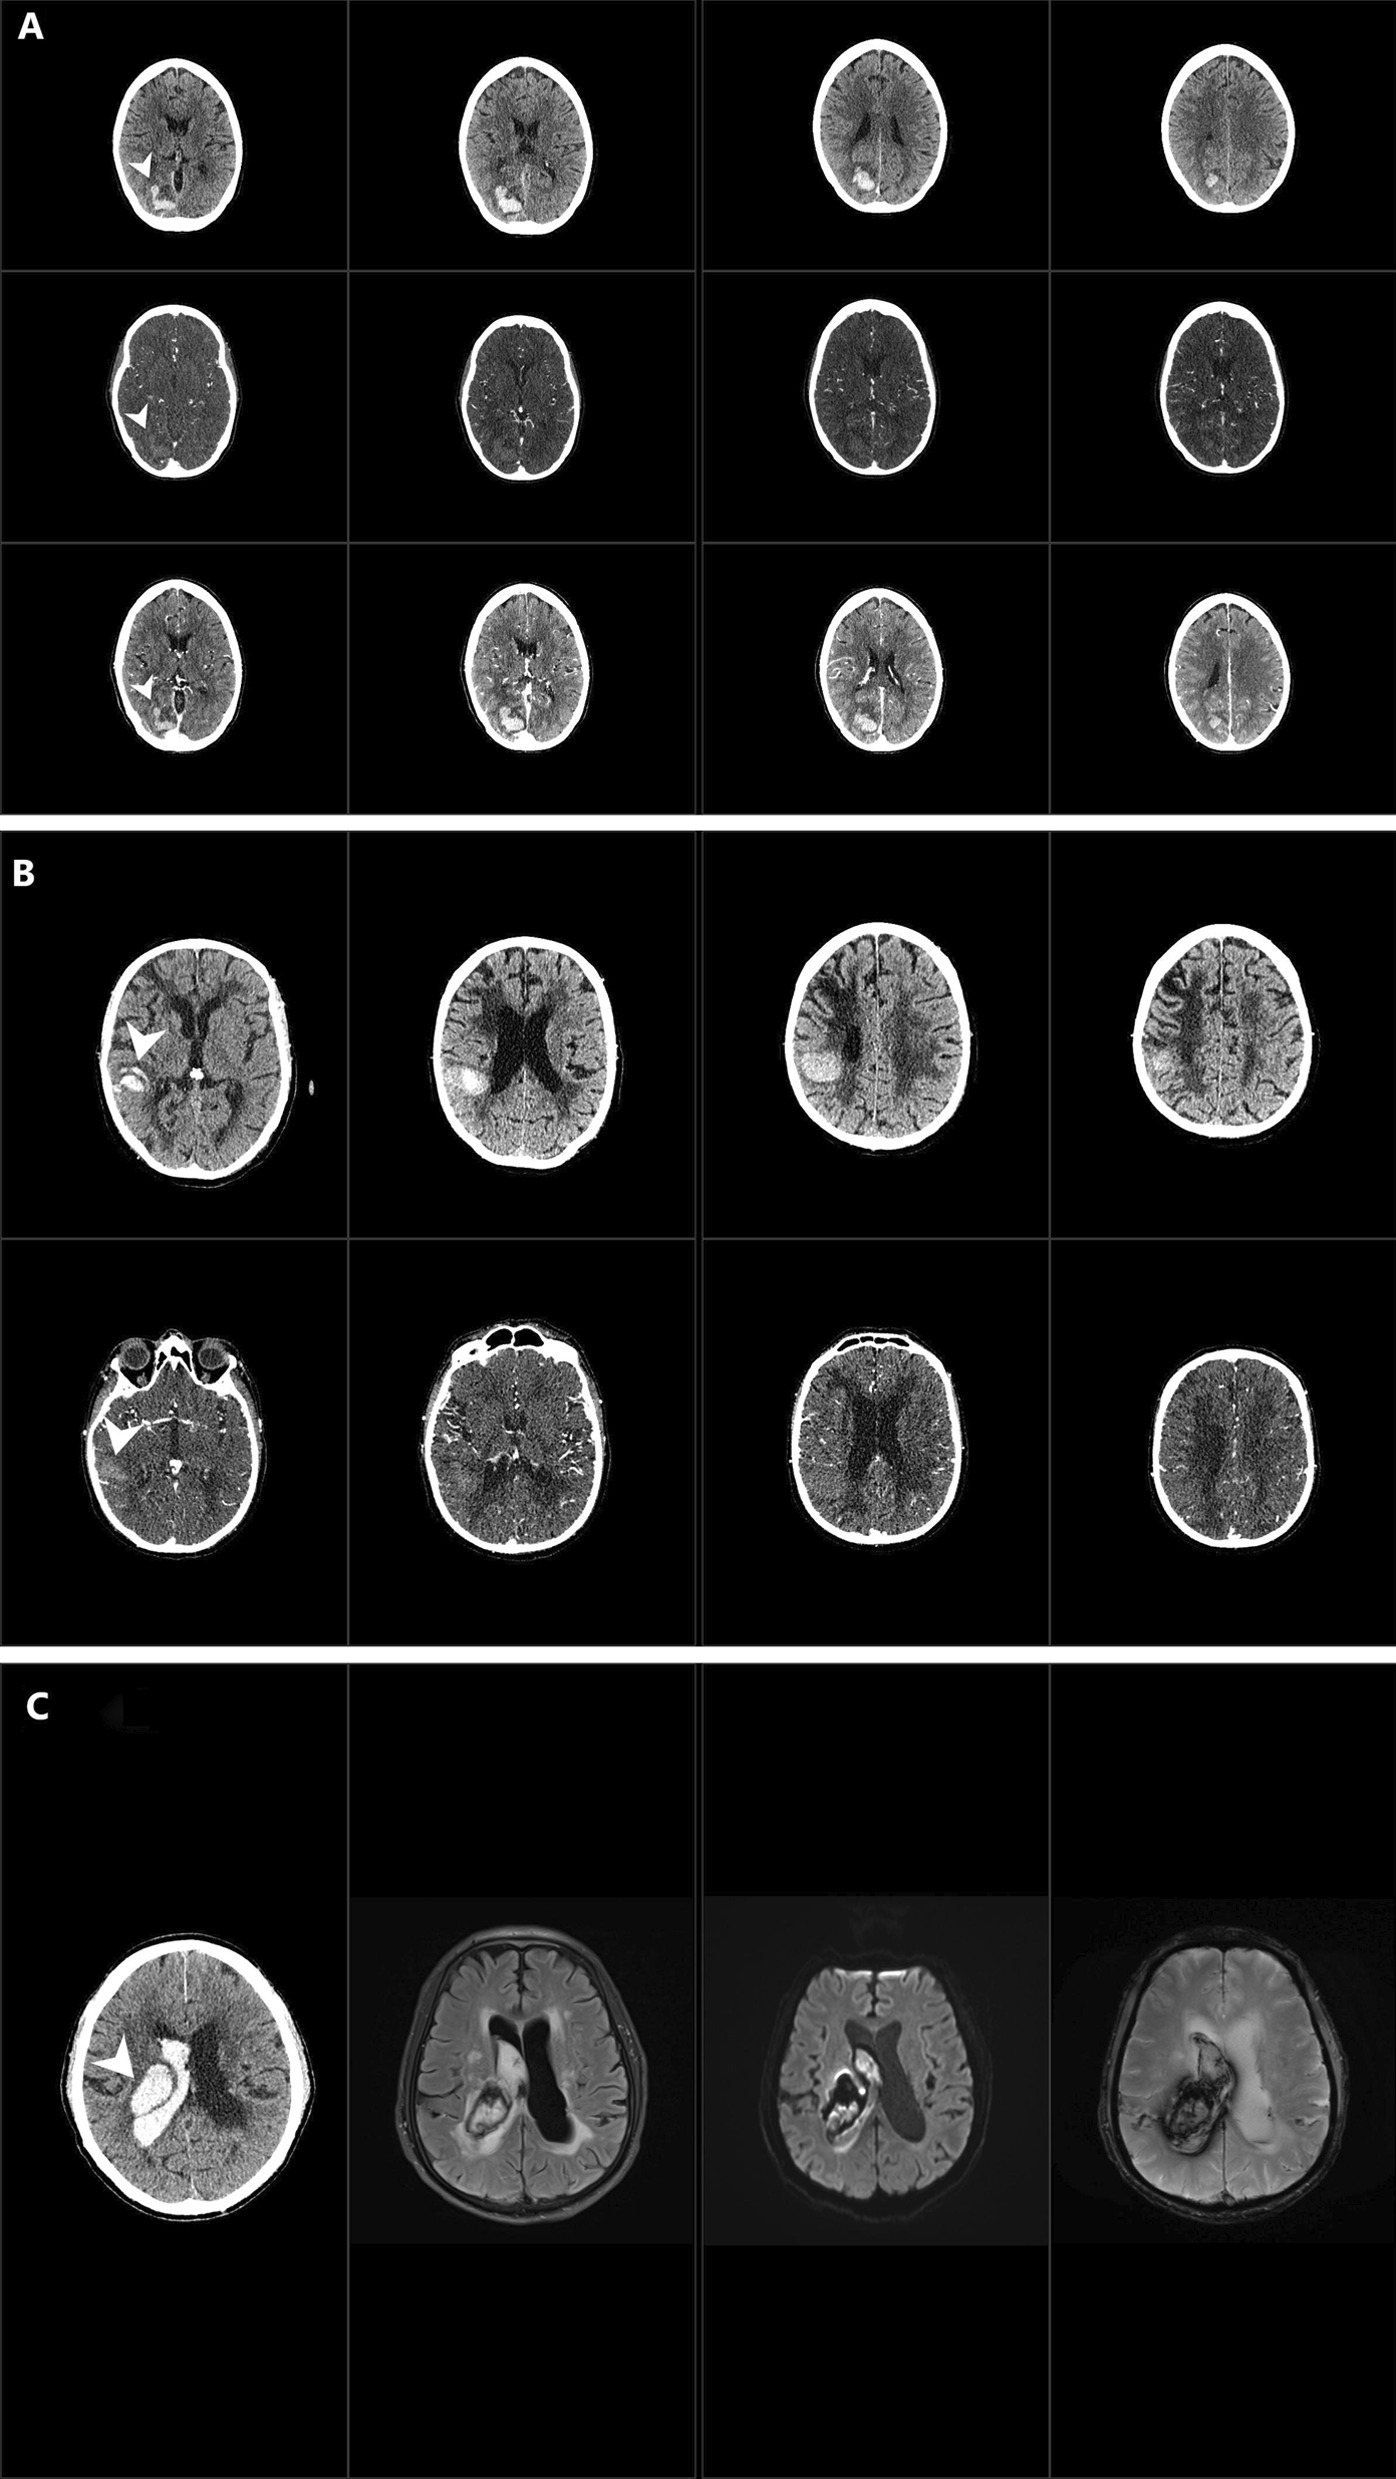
a Comparison of the same patient with ICH in the right occipital lobe (arrowhead) using different imaging modalities. The borders of the hematoma can be seen with clear contrast on admission NCCT (upper row) and follow-up postcontrast CT (lower row). On CTA (middle row) borders of the hematoma are difficult to distinguish from surrounding parenchyma. b In this example of a patient with right temporoparietal ICH (arrowhead), hematoma appears to be smaller on follow-up CTA (lower row) compared to admission NCCT (upper row). c Right thalamic ICH on NCCT, FLAIR, DWI and T2* (from left to right). On MRI sequences hemorrhage are more heterogeneous and borders are not as easily to distinguish from the surrounding tissue. Hemorrhage appears to be bigger on T2*
